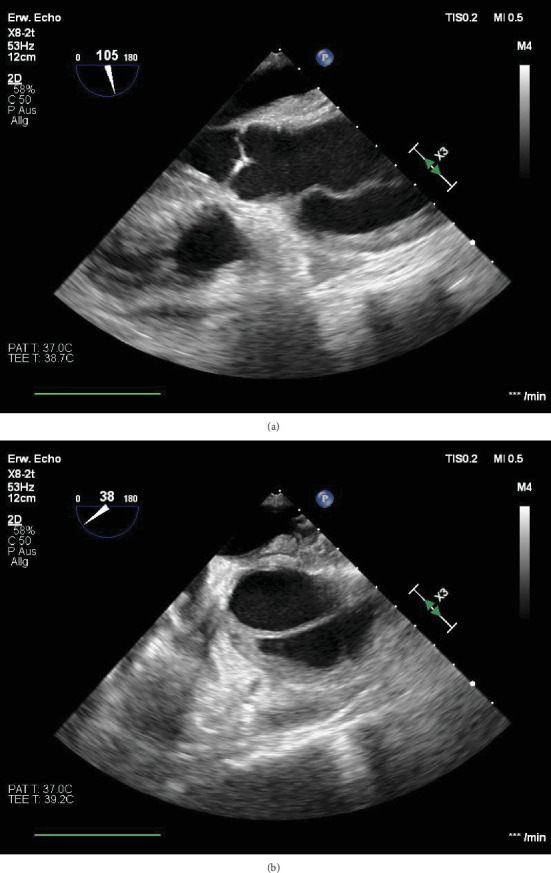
(a) Preoperative transesophageal echocardiography (TOE) of the aorta (longitudinal axis), showing distinct aortic wall thickening and the presence of a dissection membrane. (b) Preoperative transesophageal echocardiography (TOE) of the aorta (short axis), showing the same wall thickening and the dissection membrane.
